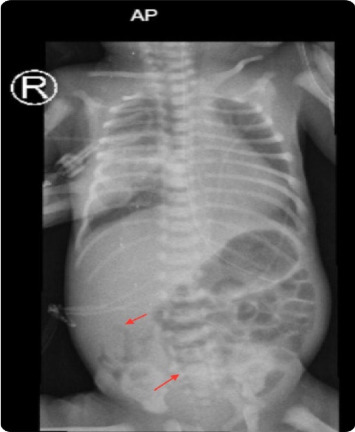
Supine abdominal X-ray showing air within bowel walls (red arrows) (pneumatosis intestinalis).
